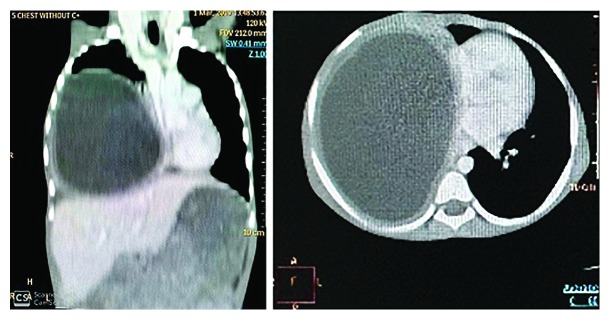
CT scan showing cyst in the right hemithorax.
